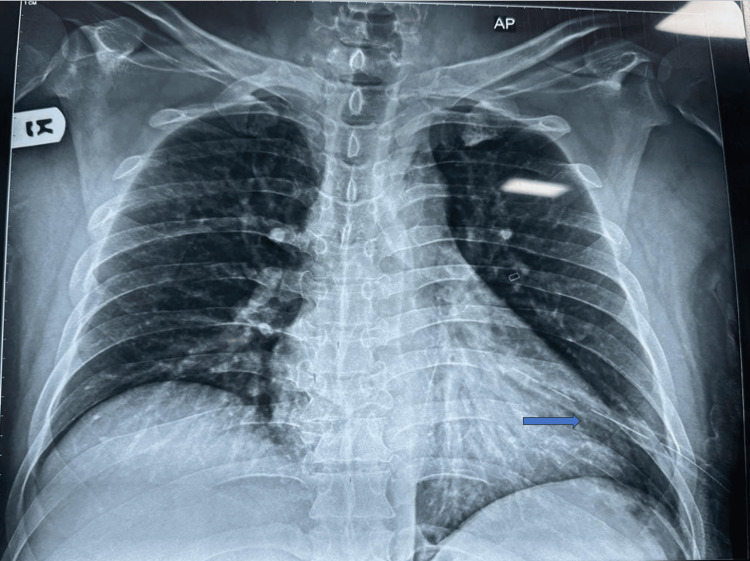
X-ray chest showing left intercostal drain (ICD) in place The blue arrow shows the intercostal drain
